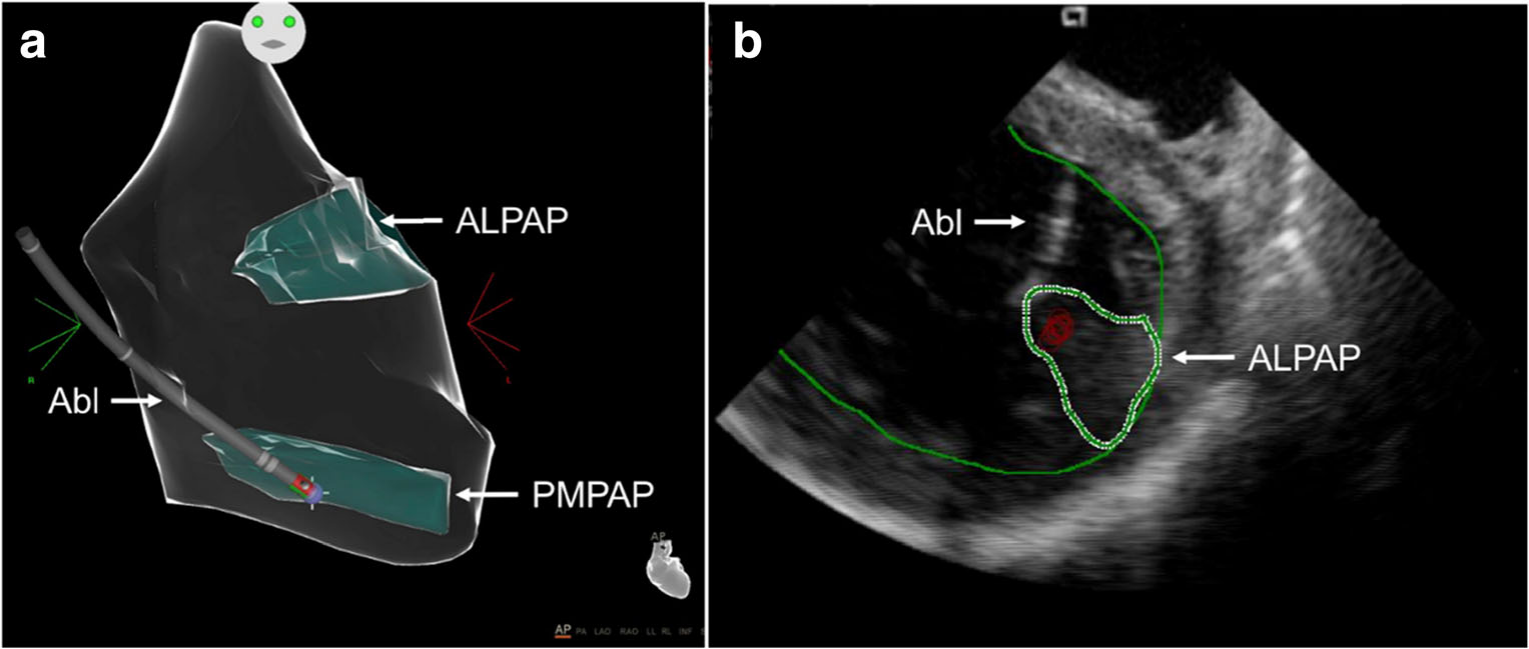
Intraprocedural imaging during ablation of papillary muscle arrhythmias. (A): Anatomical map of the left ventricle (CARTO, Biosense Webster) showing contact of the ablation catheter (Abl) with the posteromedial papillary muscle (PMPAP). (B): Intracardiac echocardiogram showing real-time visualization of the ablation catheter during ablation on the anterolateral papillary muscle (ALPAP)
